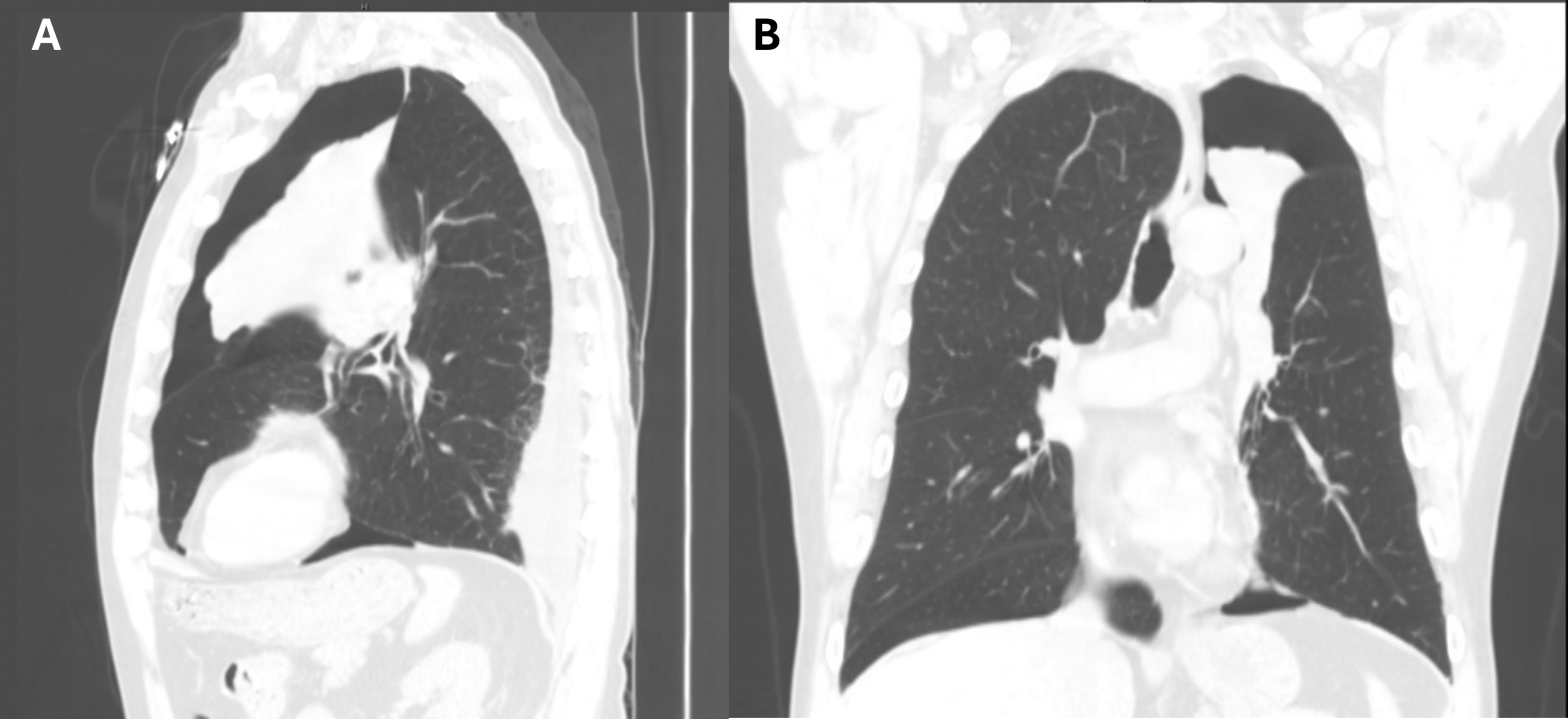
(A) Sagittal and (B) coronal CT images of patient #1 post BLVR showed complete left upper lobe atelectasis and left pneumothorax.
